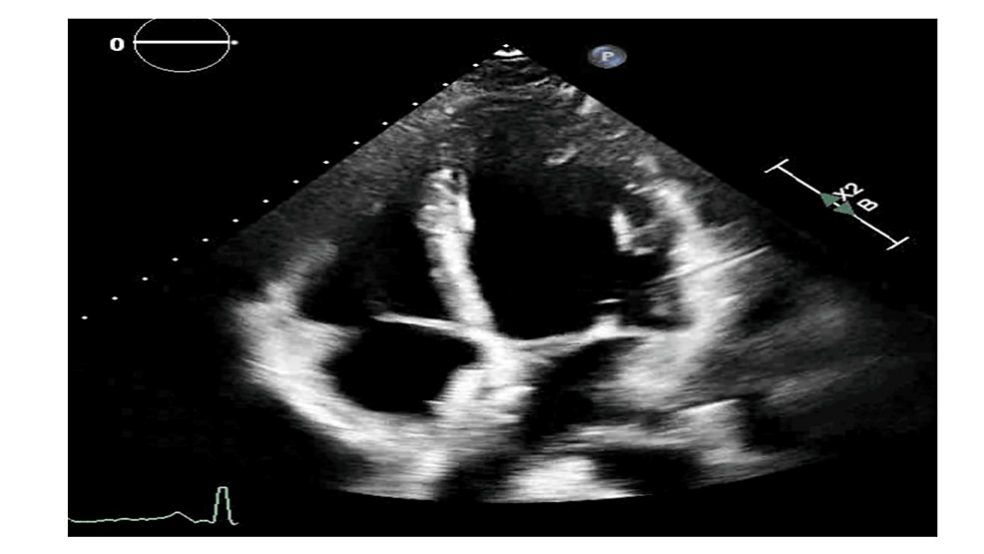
Echocardiogram after PFO closure showing occluder in adequate position traversing the atrial septum without a residual shunt and negative bubble study
